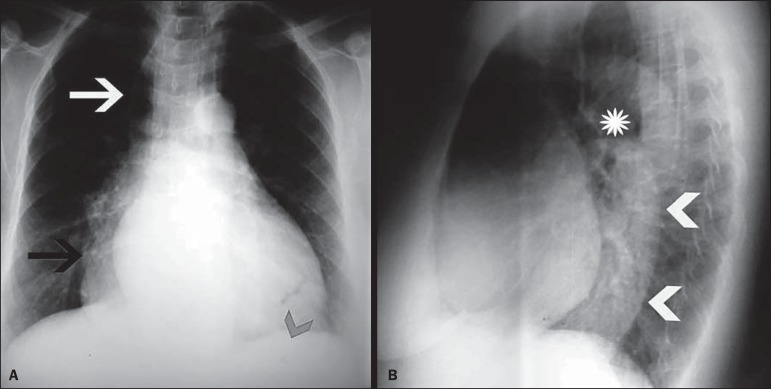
Grade III megaesophagus. A: Posteroanterior X-ray showing
